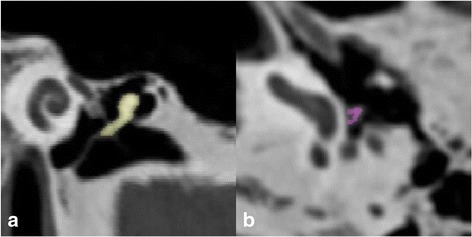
CT image-based masks. a. Mask of the malleus. b. Mask of the stapes
